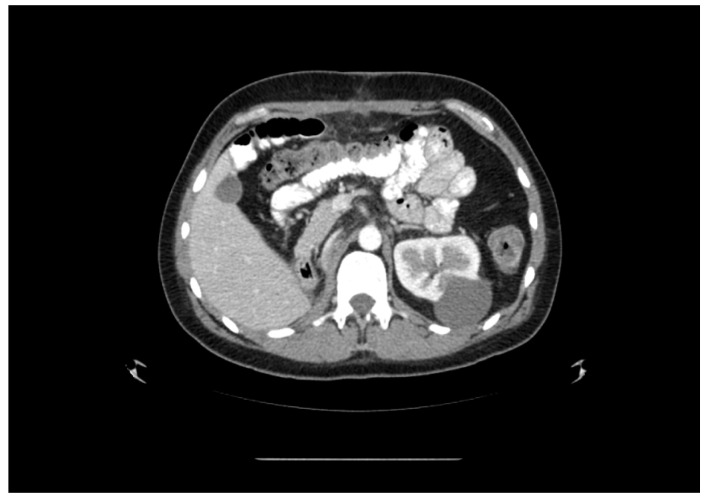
CT image. Post-right-nephrectomy view. Cyst in the left kidney is shown.
